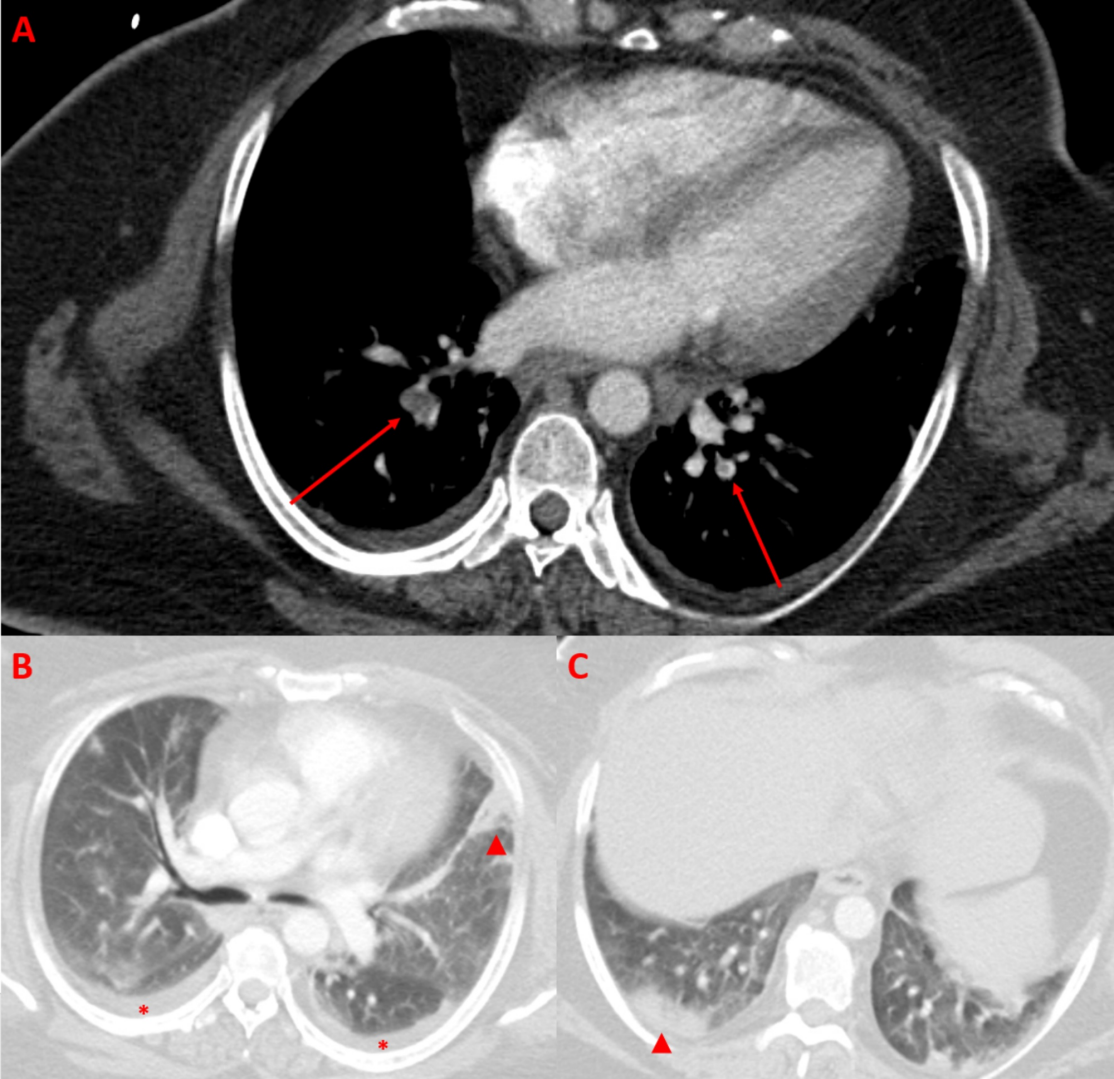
Axial CTA-PE views show filling defects in the bilateral lower lobe pulmonary arteries (arrows) representing pulmonary emboli (A). Lung windows show peripheral ground-glass and consolidative lung opacities (arrowheads). Also note bilateral pleural effusions (asterisk) (B, C). CTA-PE, CT angiogram pulmonary embolism
